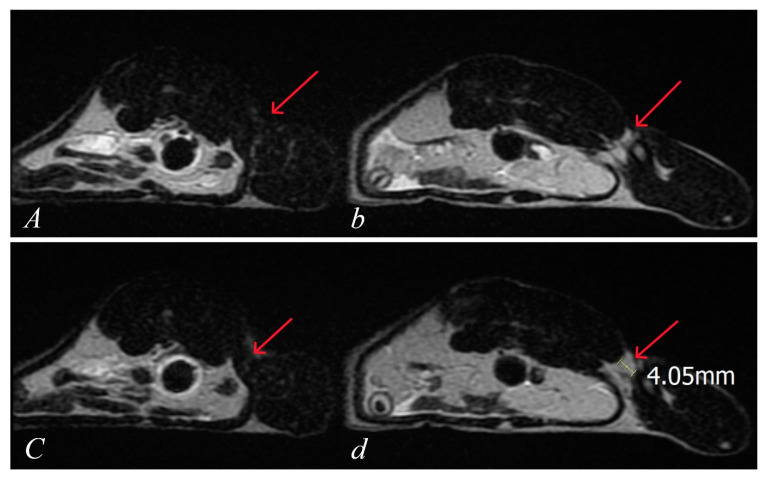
MRI imaging and measurements. Axial (*A*,*b*
